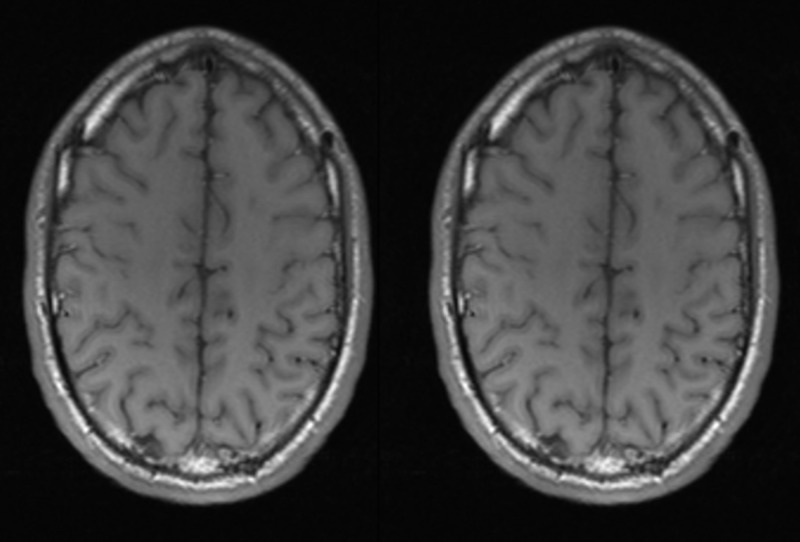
Brain magnetic resonance imaging (MRI) follow-up at four months. Brain MRI T1 Axial images showing resolution of the previously seen focal restriction and vasogenic edema.
